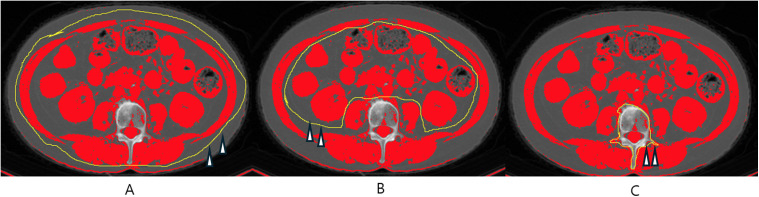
Transverse abdomen computed tomography (CT) images at the level of the third lumbar spine using ImageJ software. The threshold for the skeletal muscle of the CT scan ranges from −29 to + 150 HU. (A) Area (cm^2^) of yellow marking (arrowheads) along the outer line of the skeletal muscle mass. (B) Area (cm^2^) of yellow marking (arrowheads) along the Inner line of the skeletal muscle mass, (C) Area (cm^2^) of yellow marking (arrowheads) along the outer line of L3 vertebral body. The skeletal muscle area (SMA, cm^2^) was obtained by (A) –(C). The skeletal muscle density (SMD, HU) measured on SMA, was described as the mean HU using ImageJ software.
